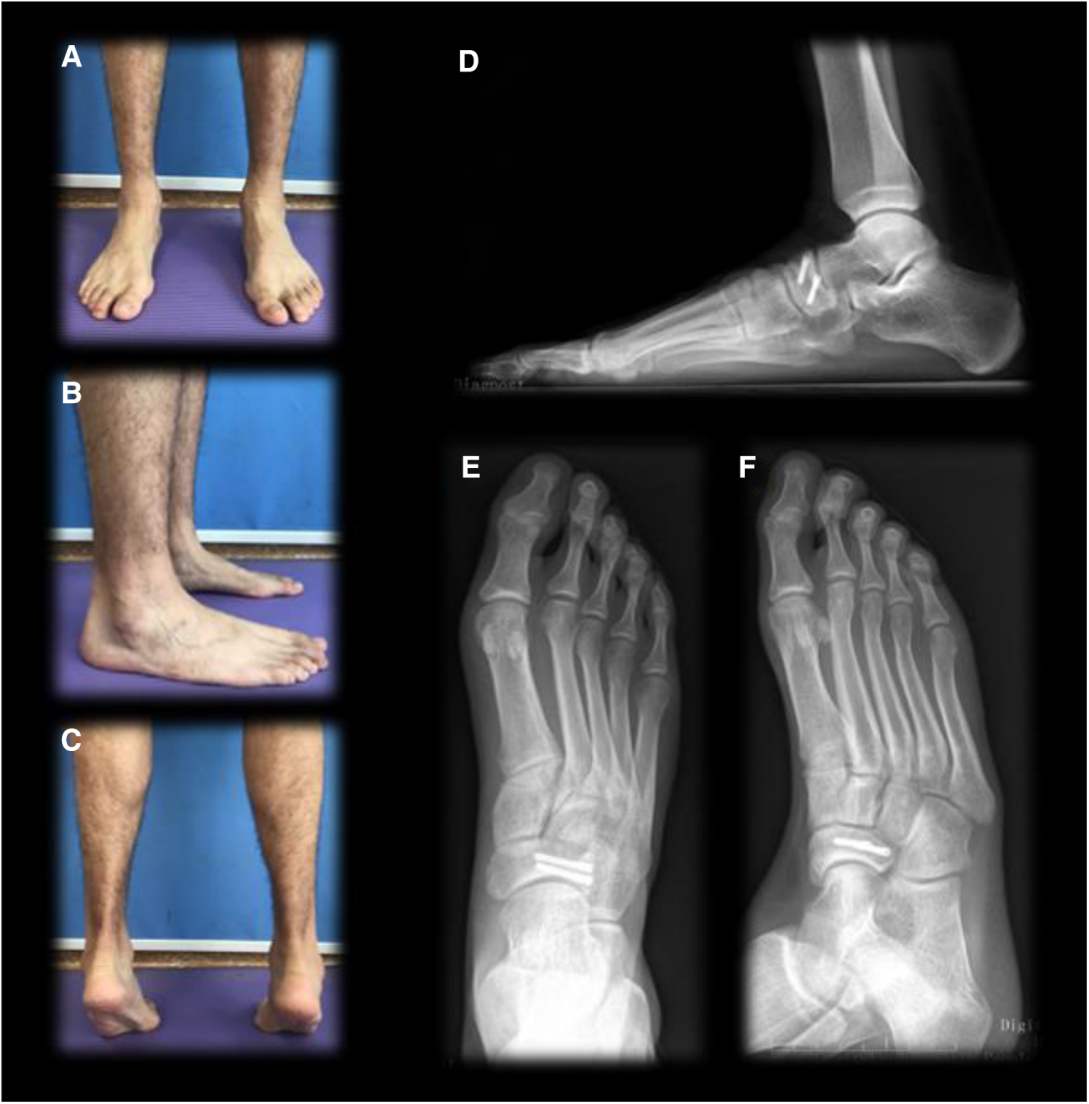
One year postoperative follow-up. (A) Front view standing on two feet; (B) lateral view of the affected foot standing on two feet; (C) rear view with both feet on tiptoe; (D) weight-bearing lateral x-ray of the right foot; (E) anteroposternal x-ray of the right foot; (F) oblique x-ray of the right foot.
